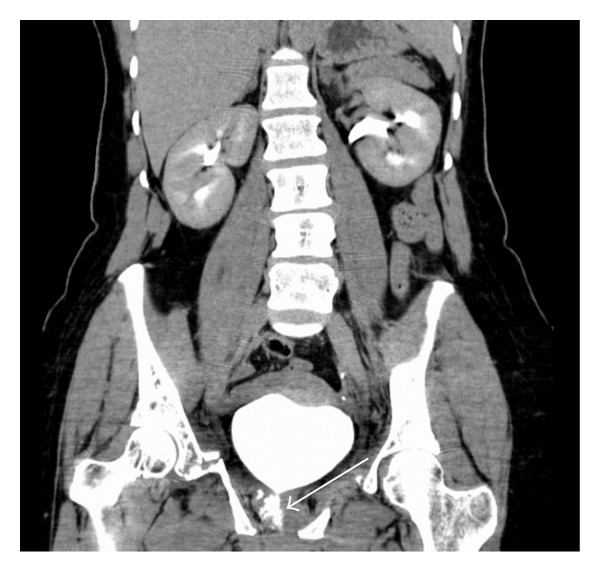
CT scan (early excretory phase) showing leakage of contrast solution from the proximal urethra into the surrounding tissues (white arrow). This typifies a urethral injury with the intact bladder.
